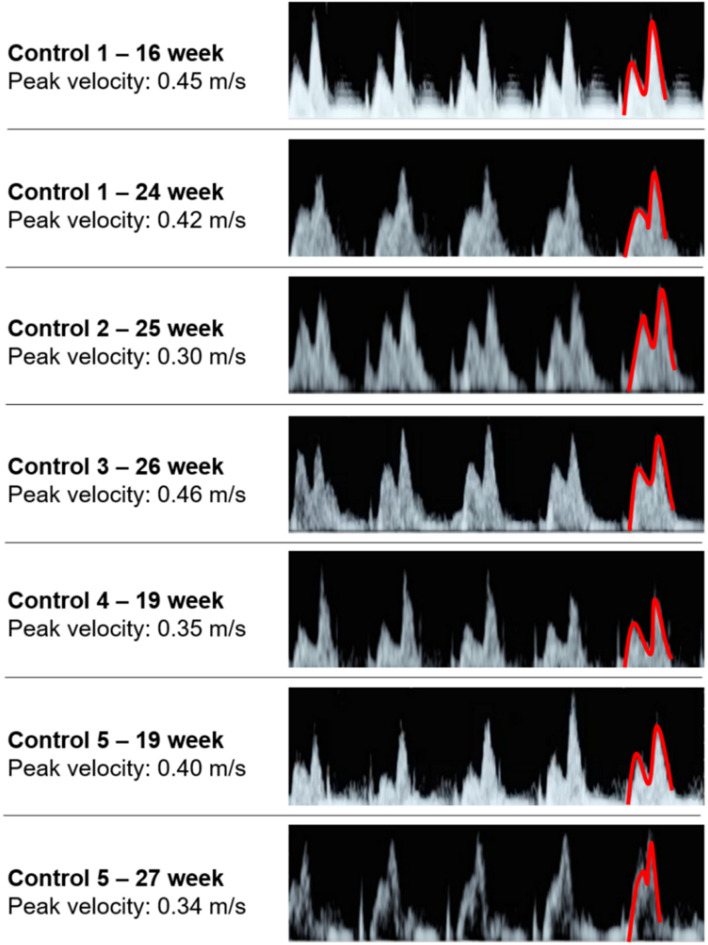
Five sequential mitral valve flow waveforms measured using Doppler ultrasound for each fetal heart. The first half of the last waveform is highlighted in red, which is showing the local maximum and peak flow velocities.
